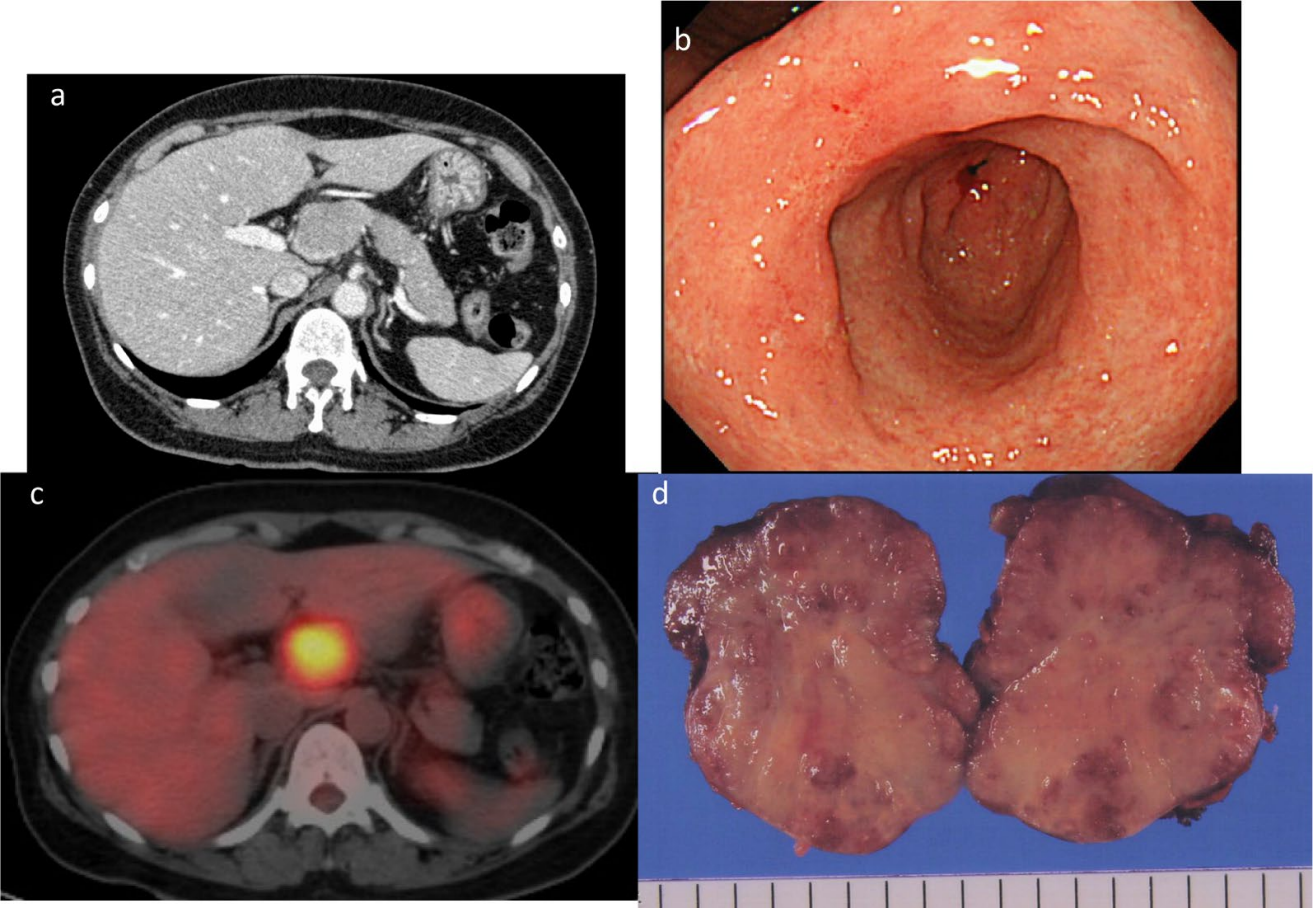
Images before lymphadenectomy. a CECT revealed the existence of a relatively uniformly enhanced nodule in contact with the upper edge of the pancreatic head. b Gastroscopy showed a small ulcer scar at the angle of stomach. c PET showed the accumulation of F-18-FDG only in the suprapancreatic nodule, as demonstrated by CECT (maximum standardized uptake value: 5.83). d Macroscopic findings from the resected lymph node showed solid, elastic, soft, and proliferation in an expansive manner (size: 51 × 42 × 31 mm). *CECT* contrast-enhanced abdominal computed tomography, *F-18-FDG* fluorine-18-fluorodeoxyglucose, *PET* positron emission tomography
